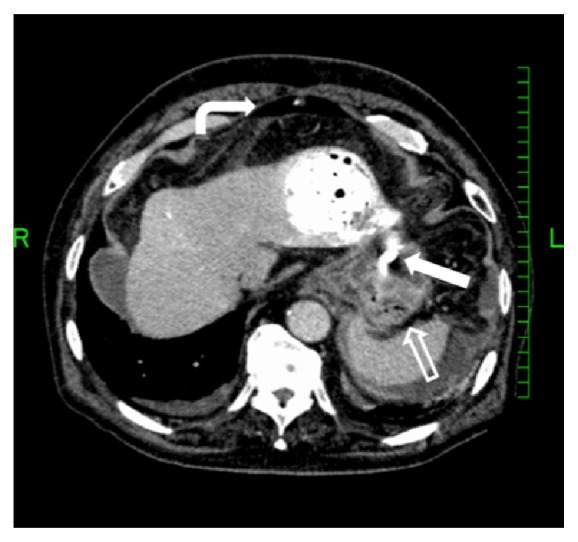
A contrast enhanced computed tomography scan of the abdomen showed iodized oil (arrow) and intraperitoneal free (curved arrow) air with a rupture of the HCC in segment II into the peritoneal cavity adjacent to the gastric wall (open arrow).
